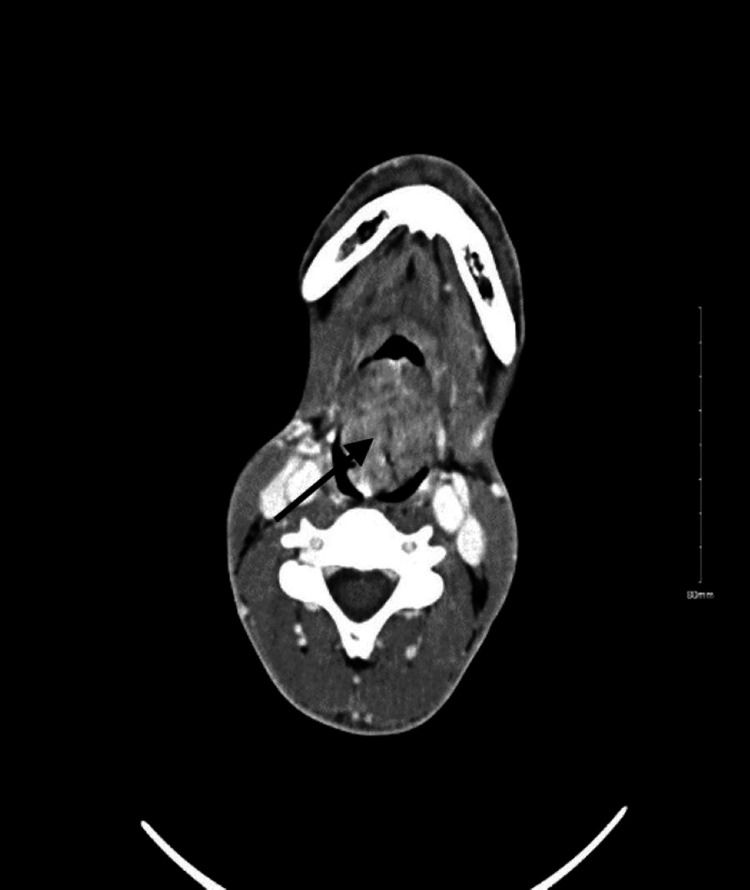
Contrast-enhanced computed tomography images (transverse view) showing a lobulated mass arising from the epiglottis and extending to the oro- and hypopharynx. The mass lesion causes near-complete obstruction of the upper airway.
